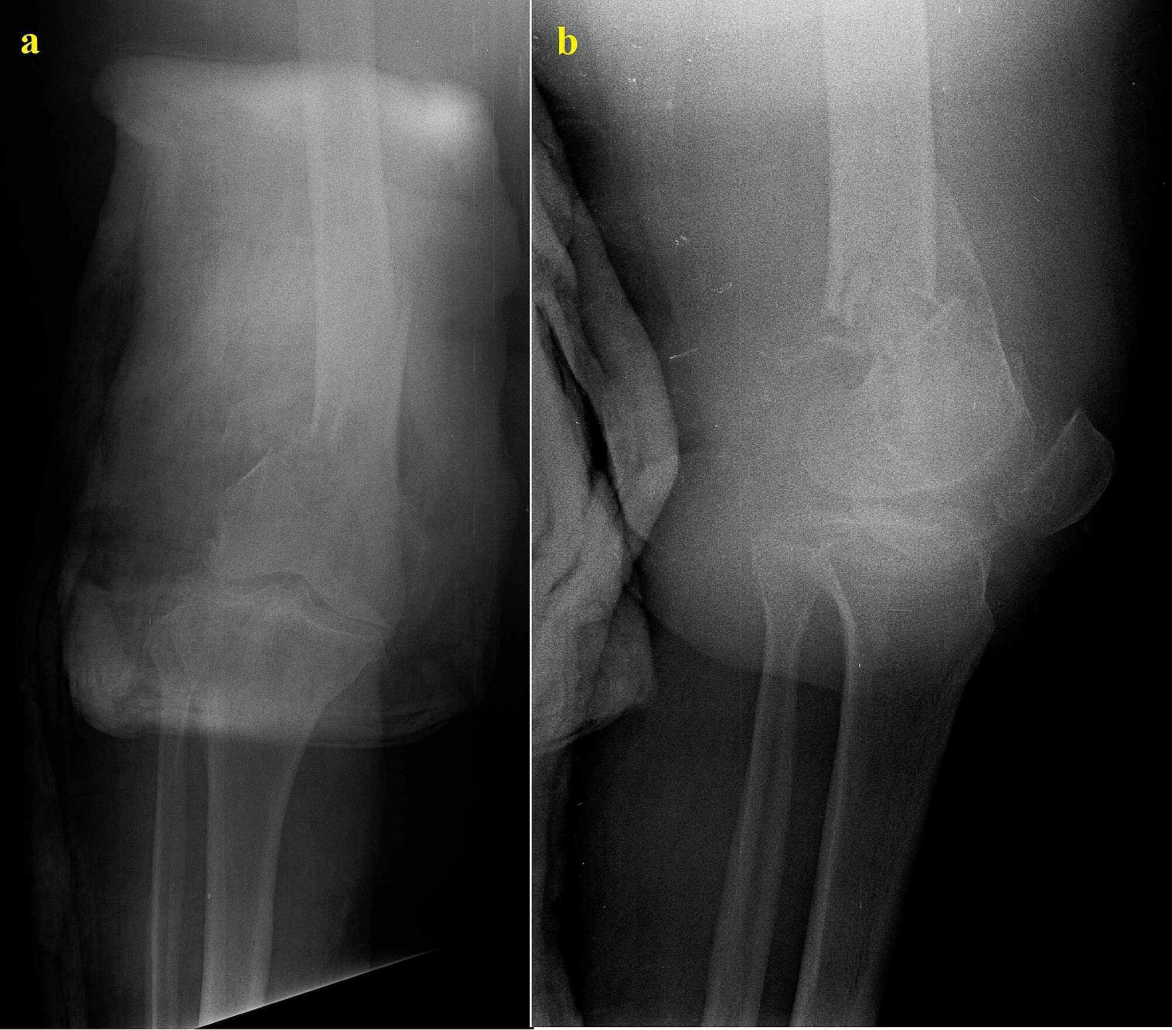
Initial anteroposterior (a) and lateral (b) radiographs of the patient showing the distal femoral fracture At closer observation, the cystic/lytic lesion can already be seen
